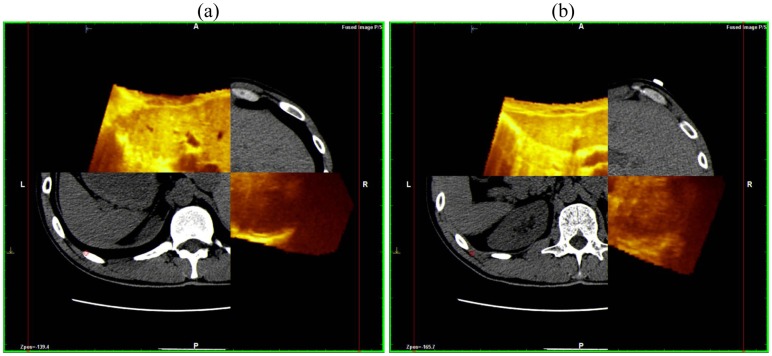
Fusion technique with NAVIGATOR system: Two different planes of reconstruction ((a) and (b)) of CT and US perfectly overlap. This system is able to separate the two planes (yellow for US, grey for CT) showing the perfect alignment of the margins with each other.
